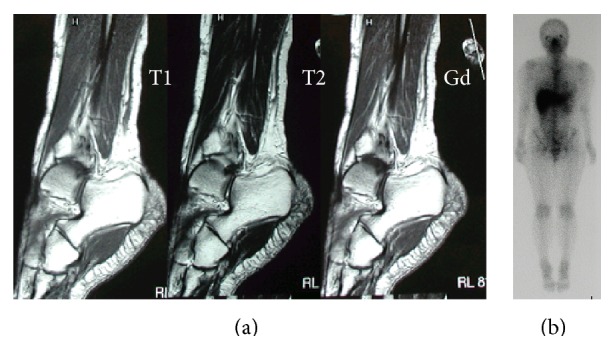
MRI (a) and bone scintigraphy (b) at 14 months after the surgery show no abnormal findings showing recurrence and local invasion of the sarcoma.
